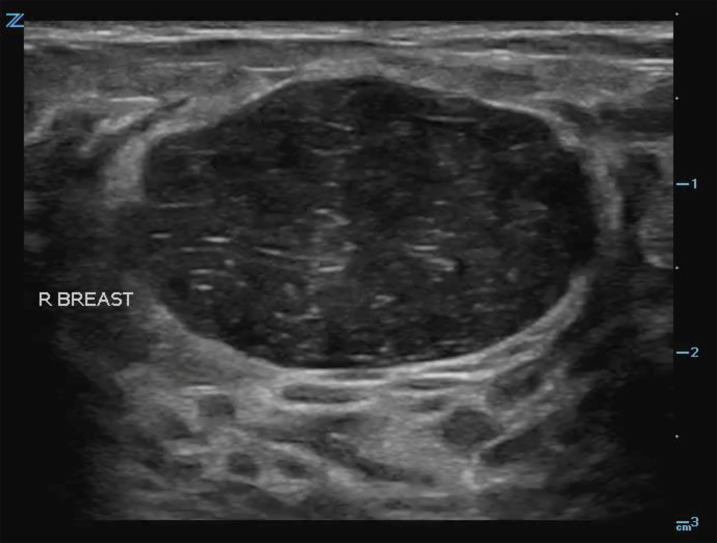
Solid, well-circumscribed mass found on a breast point-of-care ultrasound examination, later confirmed to be a fibroadenoma.
